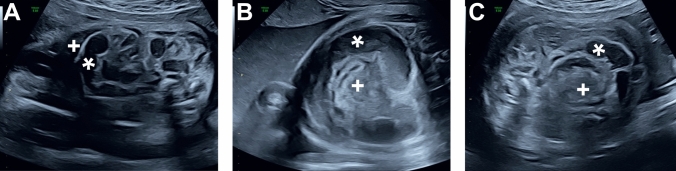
Prenatal ultrasound of a fetal volvulus. Female patient, 35 + 1 weeks of gestation. A Distended small bowel (*) and ascites (+). B Coffee bean/whirlpool sign (* and +) with distended small bowel (*). C Distended small bowel (*) and obstructed small bowel (+) due to suspected fetal volvulus
